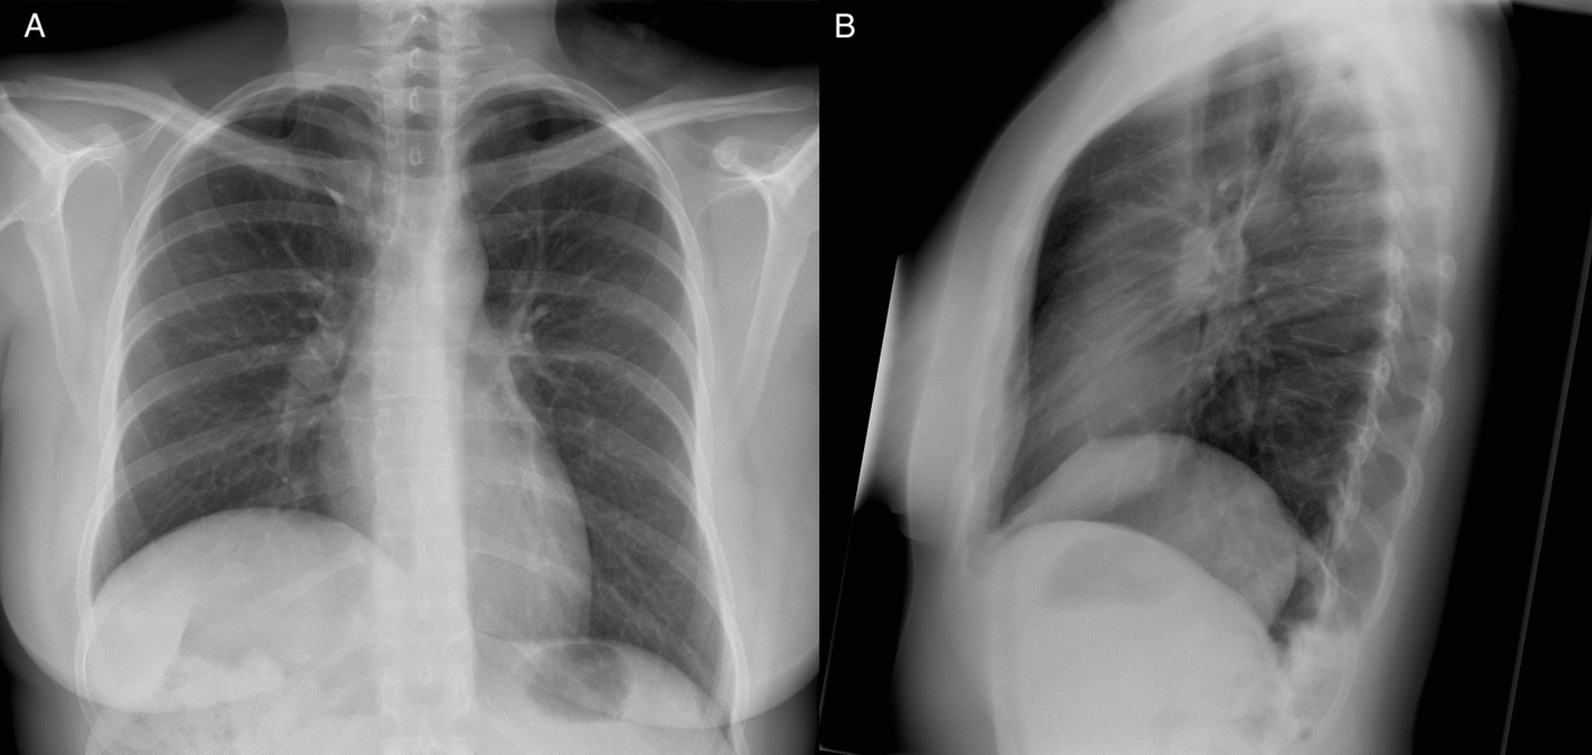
Posteroanterior (a) and lateral (b) chest X-rays demonstrating significant right-sided diaphragmatic elevation
